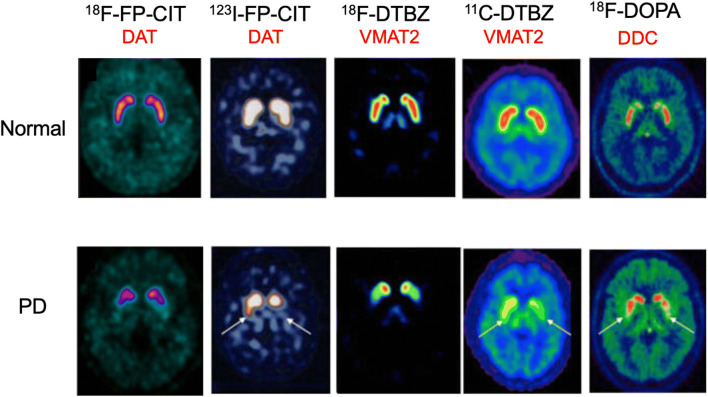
SPECT and PET images of dopamine terminal function in normal subjects and early PD patients
